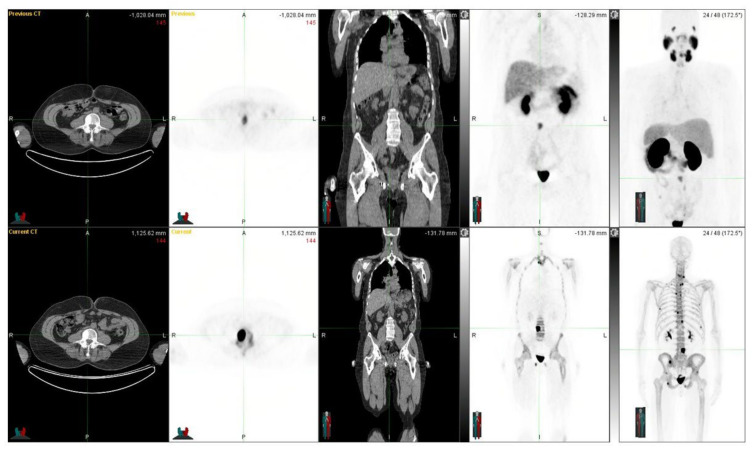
Demonstrates a situation where ^18^F-NaF PET/CT and ^68^Ga-PSMA-11 PET/CT differ significantly from each other.
